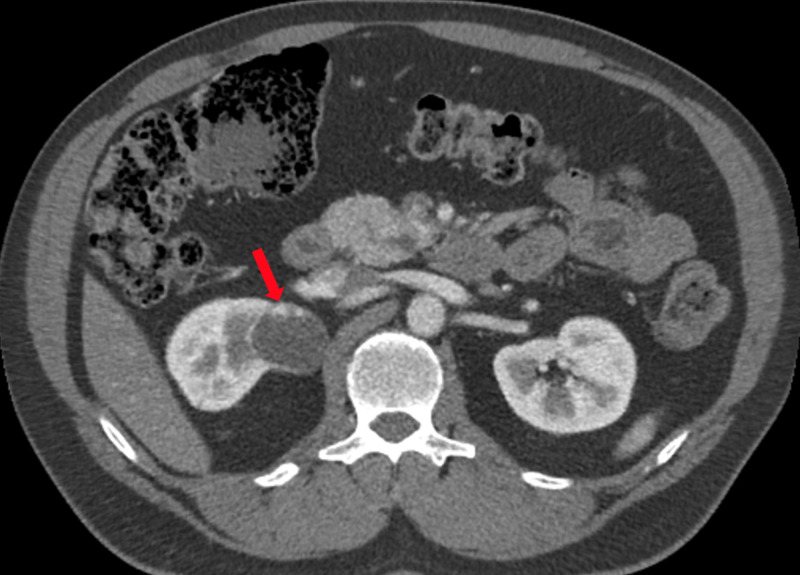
CT of the abdomen showing right renal mass (red arrow)
